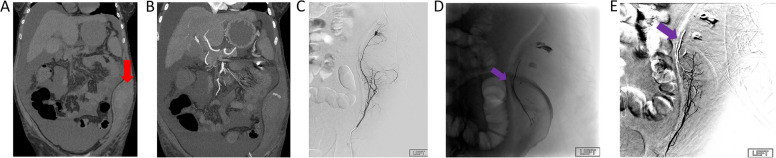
A and B pre-procedural computed tomography angiogram demonstrated left anterolateral abdominal wall hematoma and contrast extravasation (A non-contrast scan, coronal view; B arterial phase maximum intensity projection, coronal view; red arrow: hematoma and active bleeding). C selective catheter angiogram of the left deep circumflex iliac artery demonstrated contrast extravasation into the lateral abdominal wall soft tissues (red arrow: active bleeding). D Long segment arteries were occluded by radiopaque embolic (purple arrow: embolic). E Postembolization DSA demonstrated arrest of previously observed extravasation (purple arrow: embolic)
